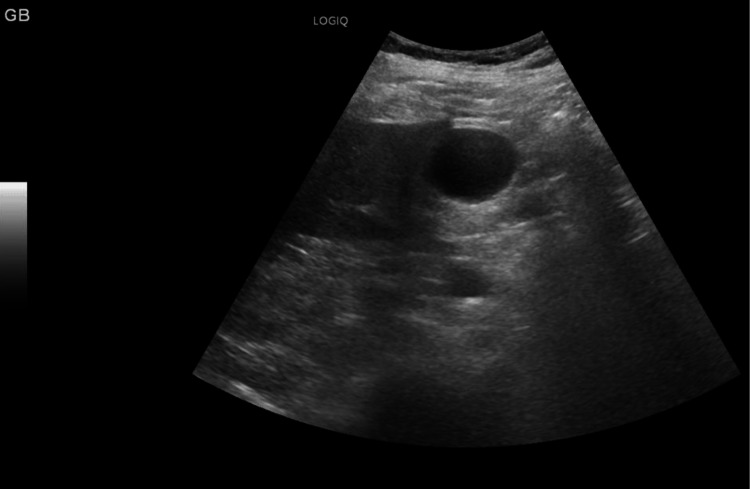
Gallbladder ultrasound. Grayscale ultrasound image demonstrating a normal gallbladder without wall thickening, gallstones, or pericholecystic fluid.
